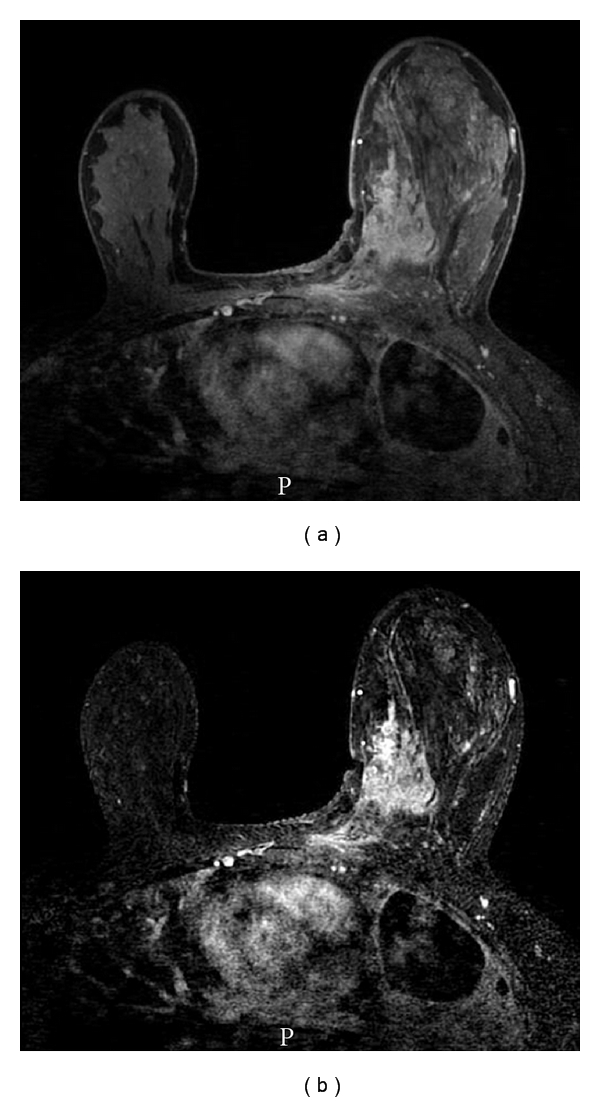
(a, b) Breast MRI demonstrated regional enhancement in the left superior breast extending to the chest wall and diffuse skin thickening.
